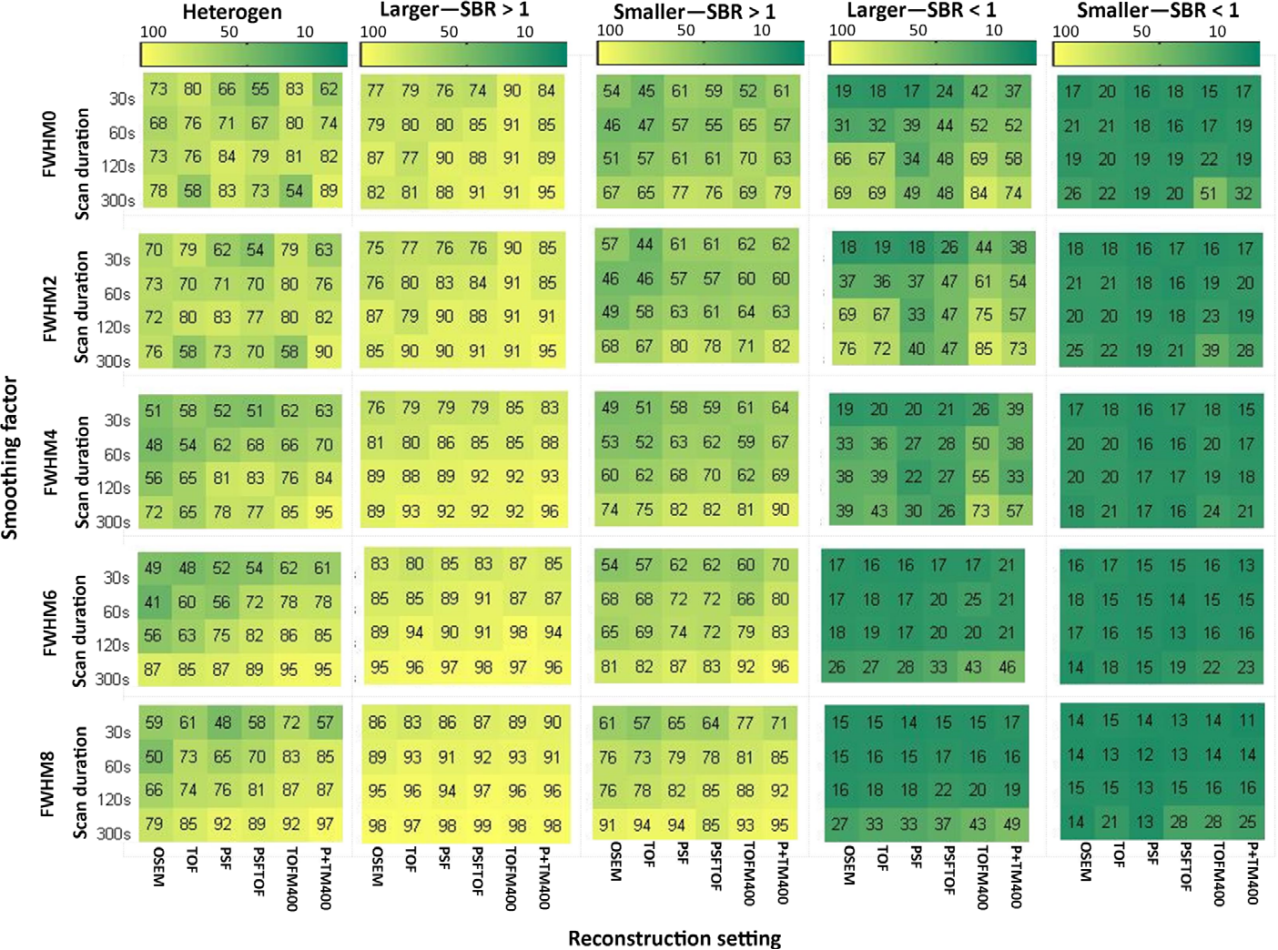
Percentage of repeatable features discretized with FBW: Percentage of all features discretized with FBW and segmented based on CT exhibiting an ICC >0.8 for all studied settings and underlying data categories (from left to right: heterogeneous 3D prints, bigger spheres with high uptake, smaller spheres with high uptake, bigger spheres with low uptake, and smaller spheres with low uptake). TOFM400/P+TM400: TOF/PSF+TOF reconstruction with matrix size 400 × 400. [Color figure can be viewed at wileyonlinelibrary.com]
